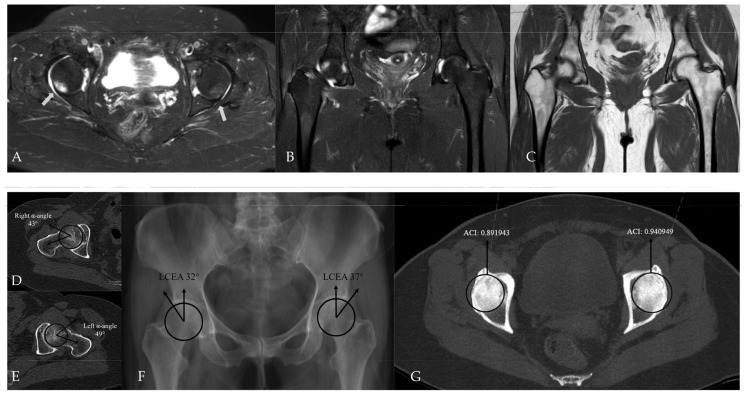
Hip MRI demonstrates bilateral posterolateral femoral head bone marrow edema on (A) axial (white arrows) and (B) coronal fat-suppressed T2-weighted images, more pronounced on the right, with associated right hip joint effusion. (C) Coronal T1-weighted image shows no structural abnormality. (D,E) CT shows normal alpha angle measurements, and (F) LCEA values are within normal limits. (G) However, the ACI exceeds the 0.93 cut-off value on the left, while CT–MIP images demonstrate posterior acetabular rim overcoverage on the right without marked posterior wall lateralization.
